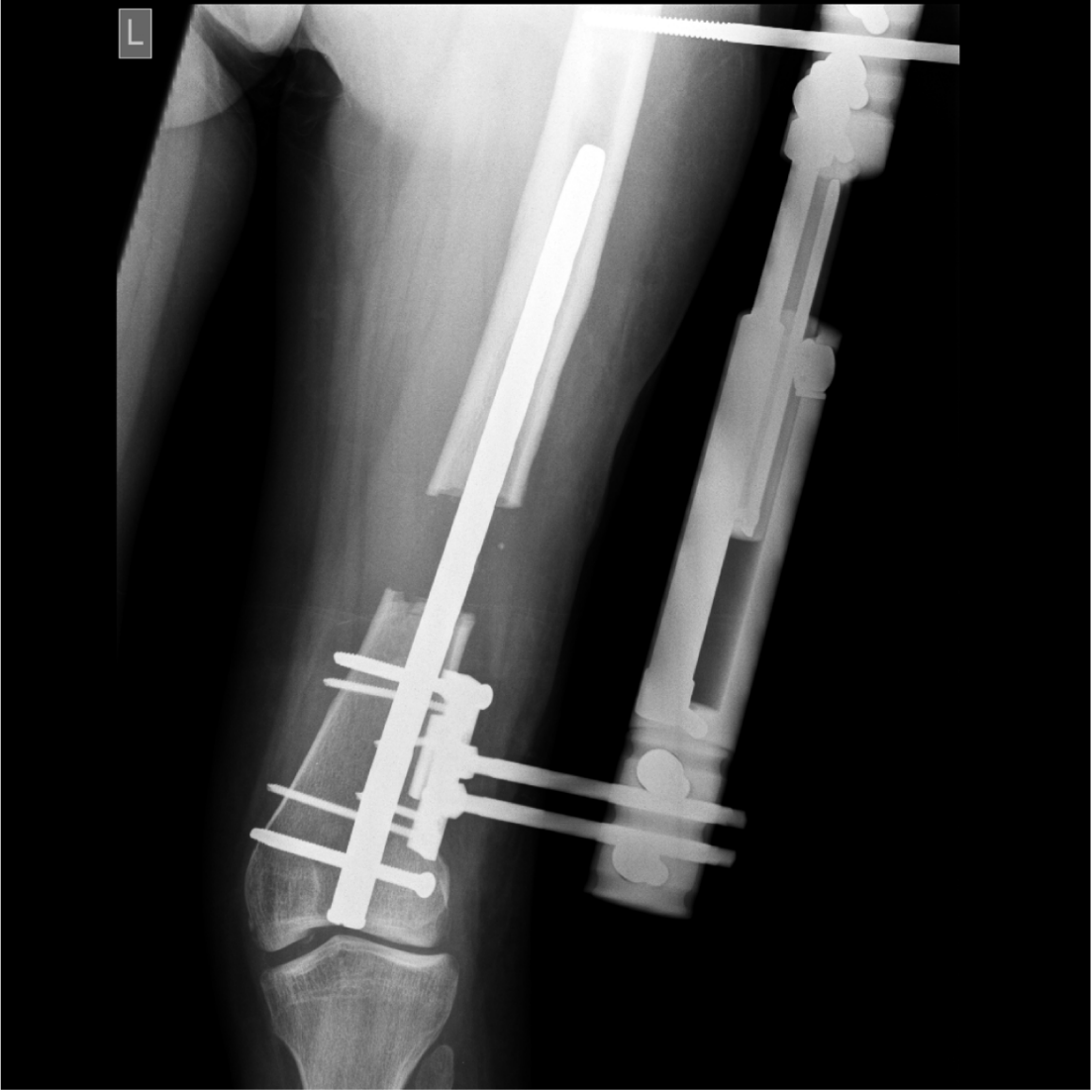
Radiograph of the distal femur in the patient from Figure 4 after the acute phase of lengthening has been completed.
